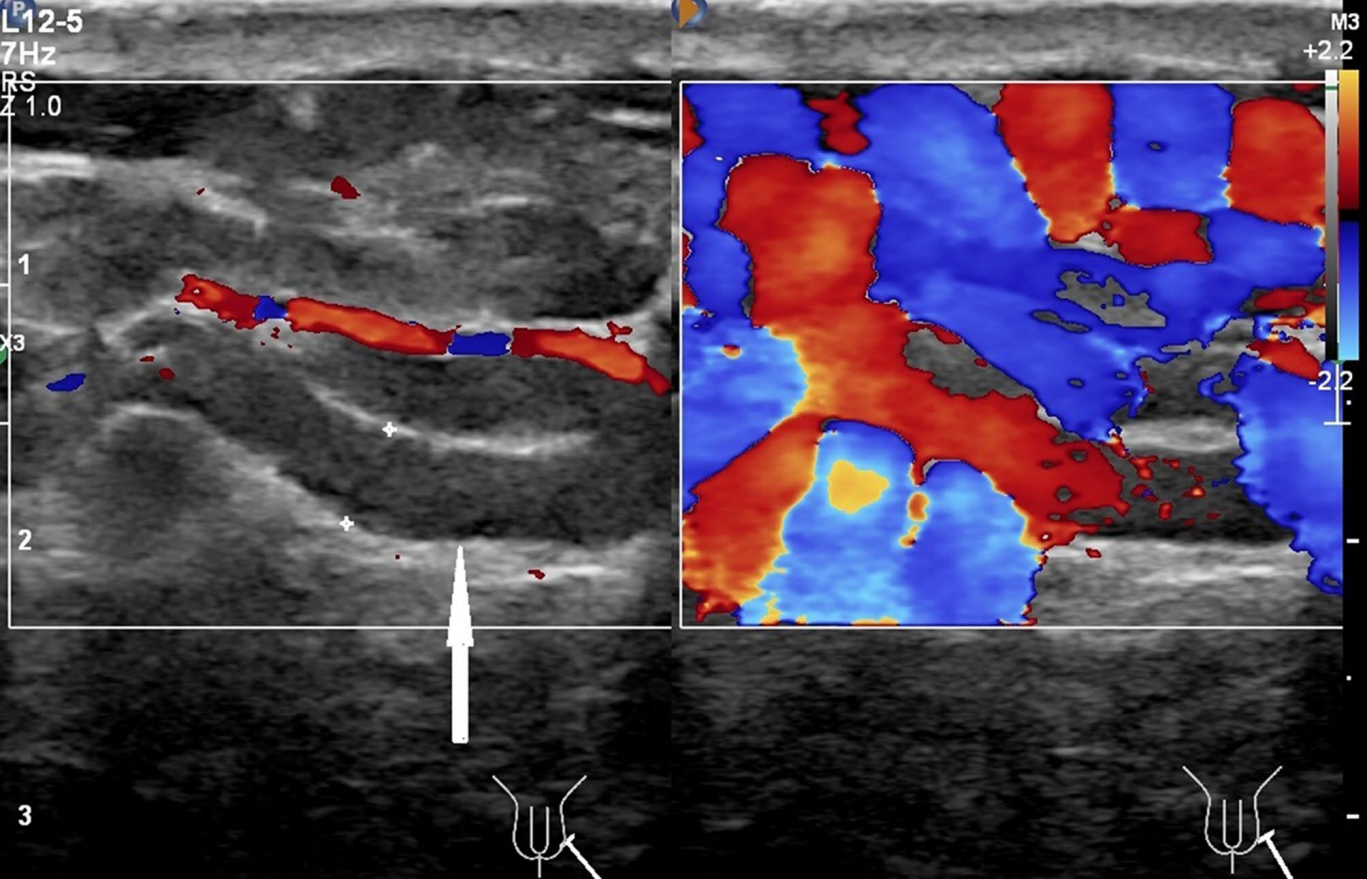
A 34-year-old man with left varicoceles. The veins of left pampiniform plexus present irregular serpiginous fluid-filled anechoic tubular structures (3.2 mm in diameter) in the region of the spermatic cord on gray-scale ultrasound at standing position (left panel, arrow); the fluid-filled anechoic tubular structures show color Doppler flow signal on duplex ultrasound (right panel).
